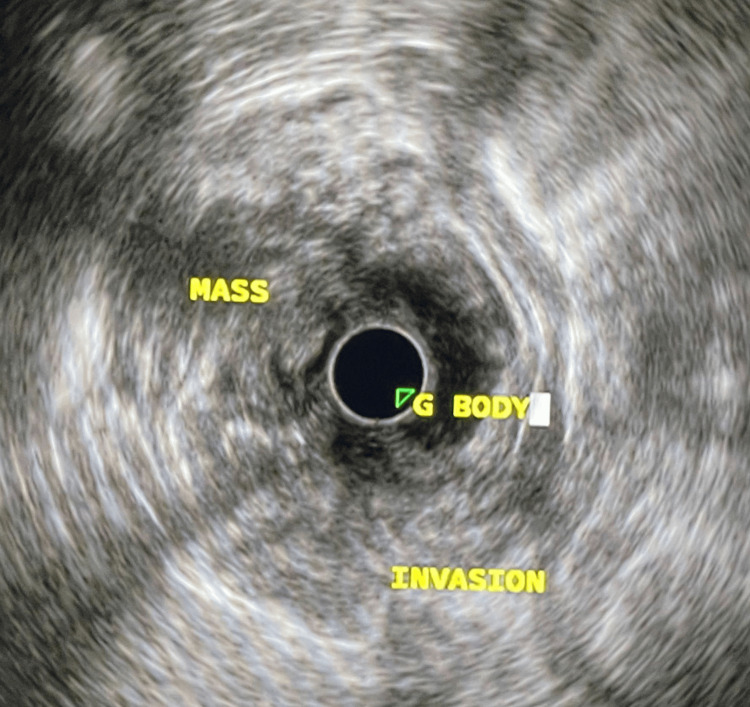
Endoscopic ultrasound showing an invasive gastric mass
